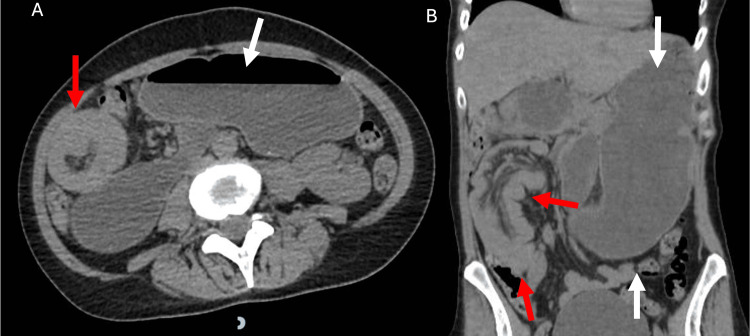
(A) Axial abdominal CT showing the target signal (red arrow) with significant liquid distension of the stomach (white arrow). (B) Coronal view of the abdominal CT with a jejunojejunal intussusception on the right side of the abdomen (red arrow) and the enormous distension of the stomach and duodenum (white arrow) CT: computed tomography
